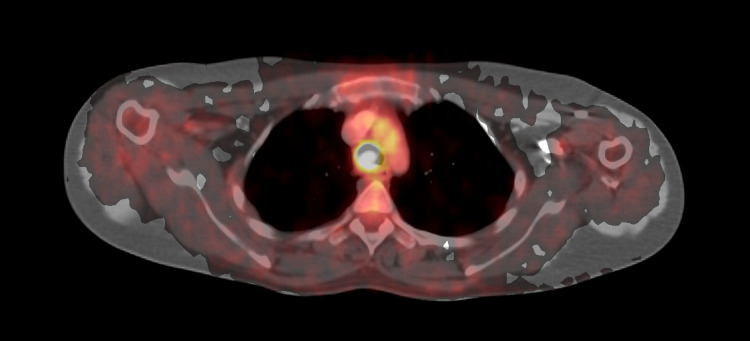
Ga-68 DOTATATE PET CT It shows a lesion of 1.3 × 1.6 × 1.5 cm with uptake of SUV max 6.3 and a minimal somatostatin receptor expression of SUV max 2.8.
